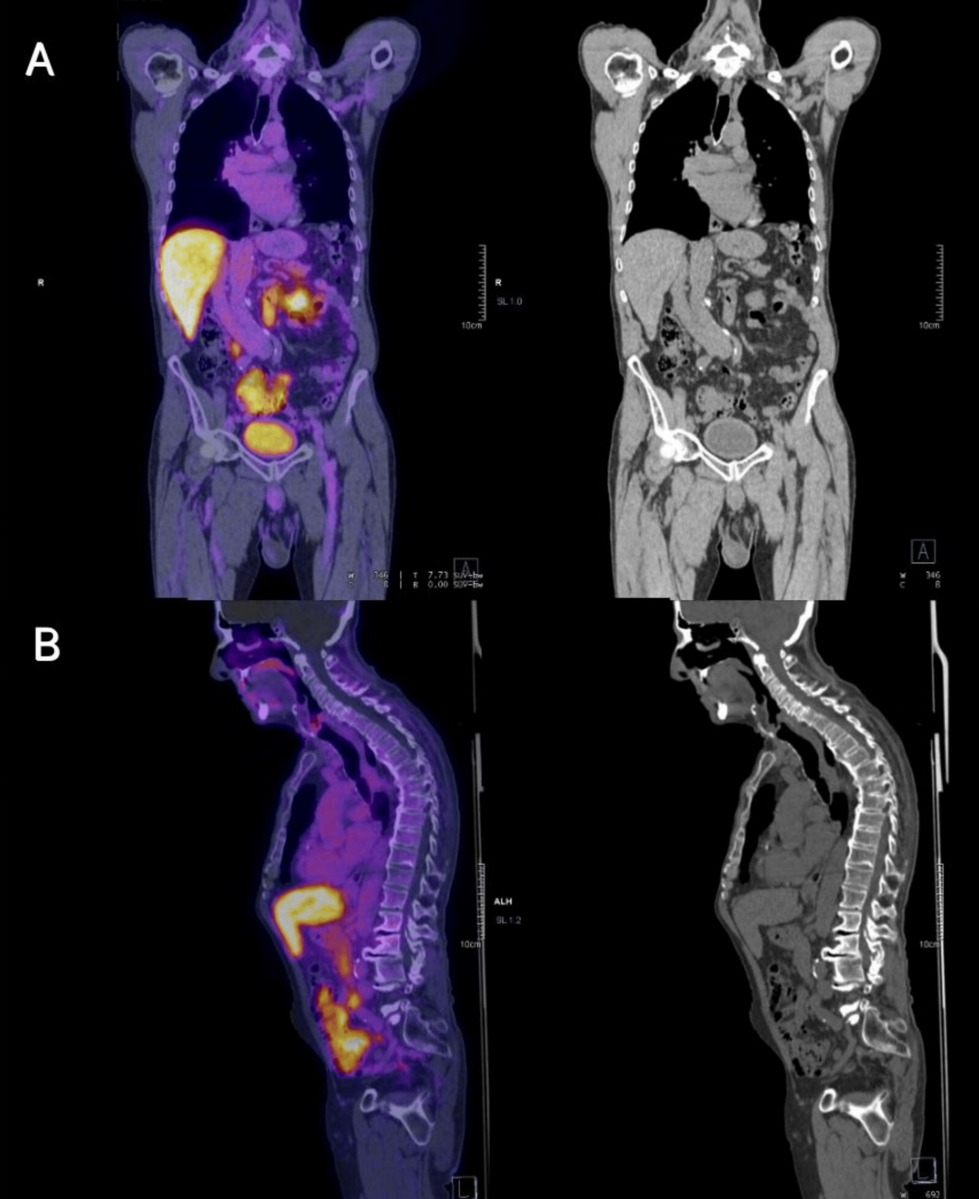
18-fluorodesoxyglucose positron emission tomography-computed tomography (18 FDG PET-CT) (A) Coronal view without evidence of increased anormal metabolism compatible with neoplastic activity. (B) Sagittal view without evidence of increased anormal metabolism compatible with neoplastic activity.
